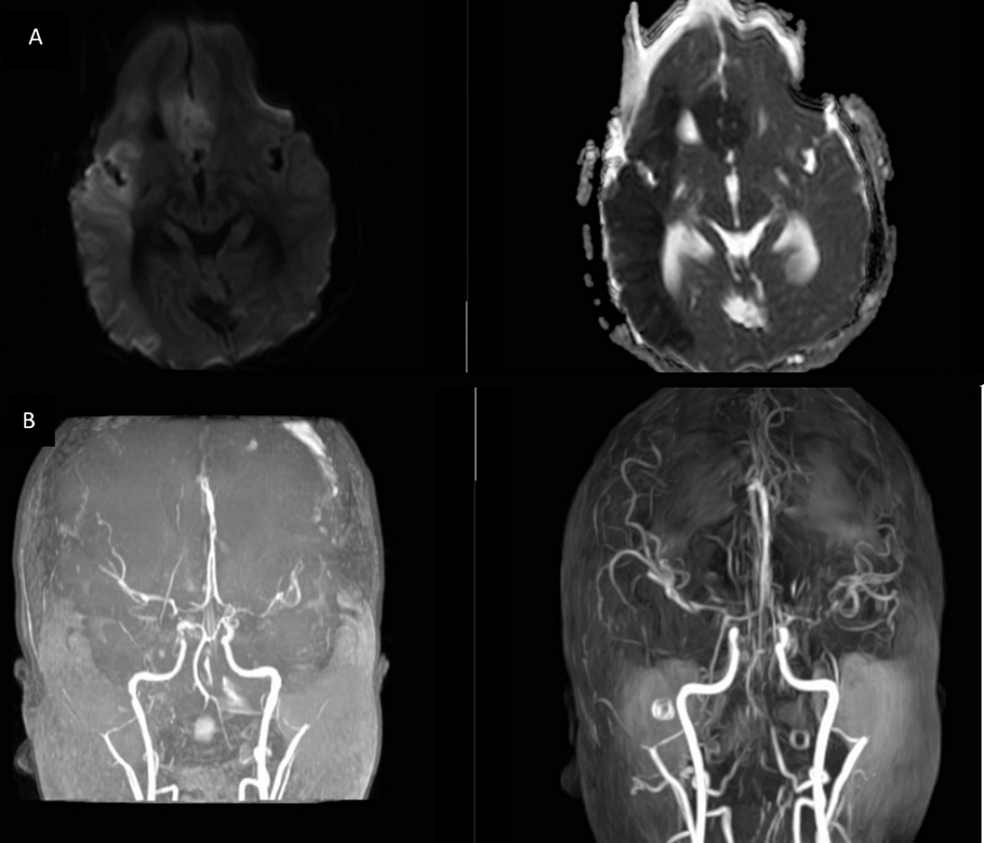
(A) Diffusion-weighted MRI of the brain showing bilateral brain ischemia. (B) Magnetic resonance angiography showing bilateral supraclinoid internal carotid artery and distal bilateral vasospasm
